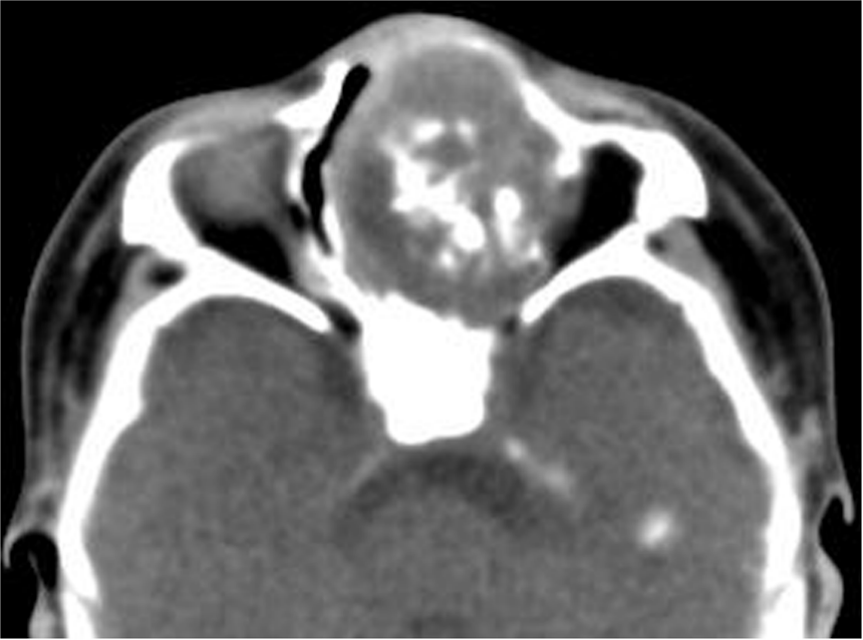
An axial non-contrast CT revealed an well-defined, irregular, calcified soft-tissue mass in the left nasal cavity.
